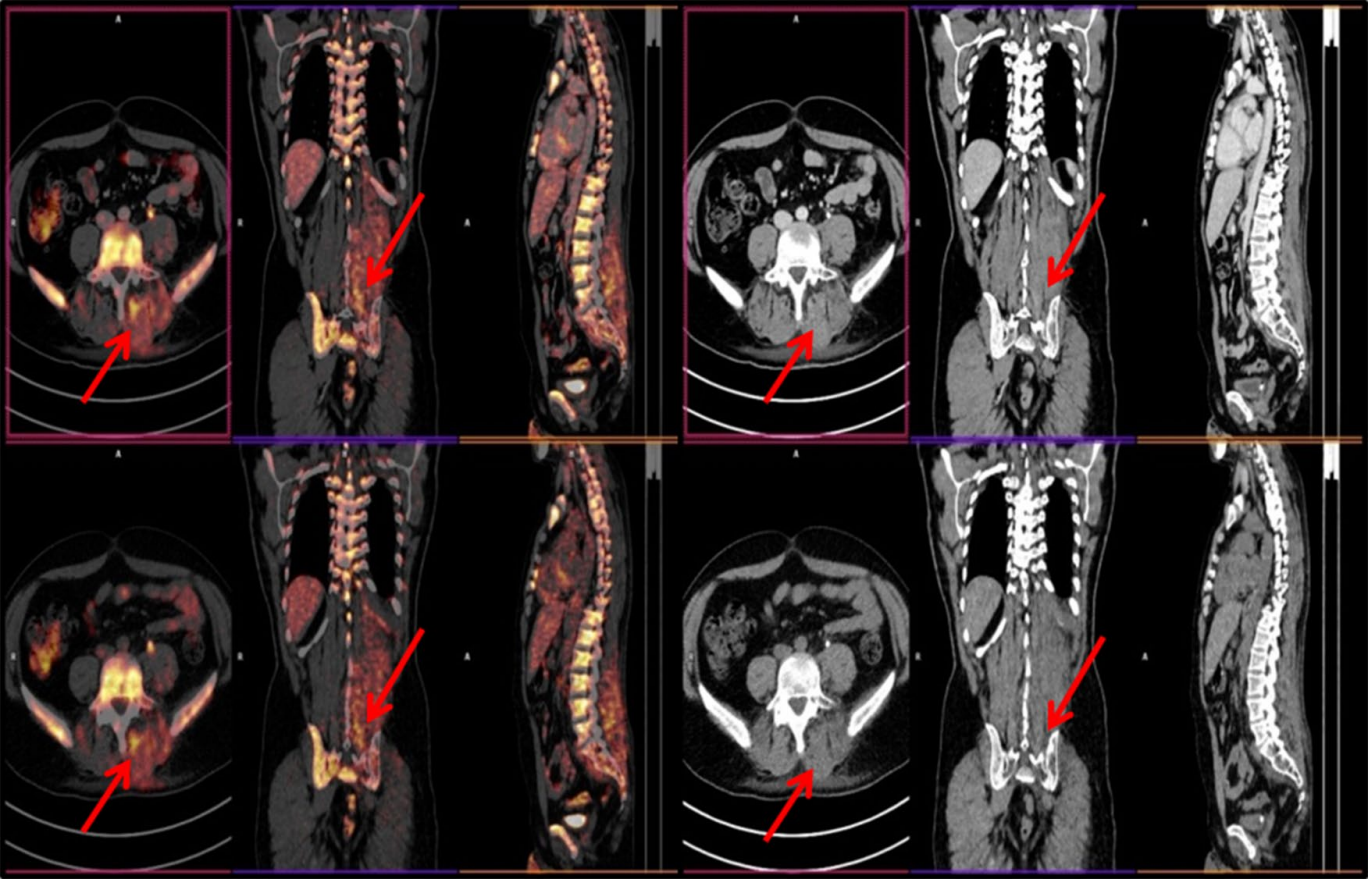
PET/ldCT and PET/ceCT showed paravertebral tissue infiltration [DS 5; SUVmax 4.7].
